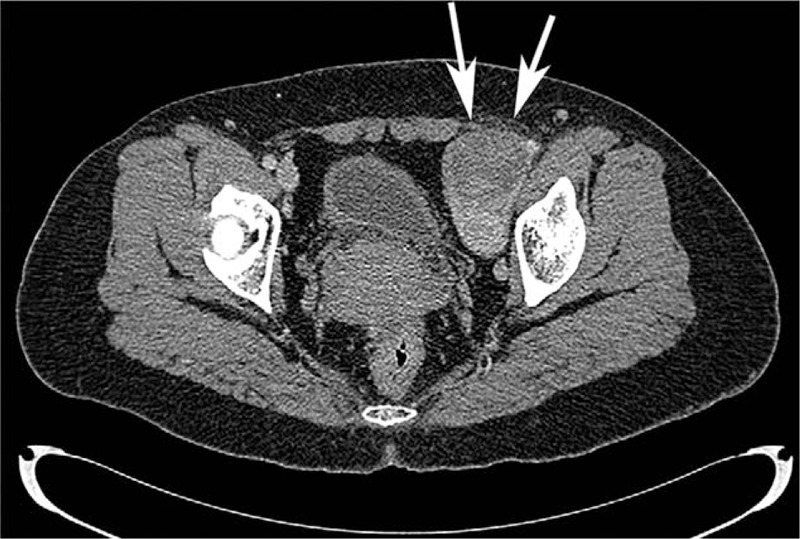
Axial contrast-enhanced venous phase CT showed an enlargement of her left external iliac vein due to a heterogeneous enhanced mass measuring7.5 × 6.0 × 5.7 cm (arrows).
